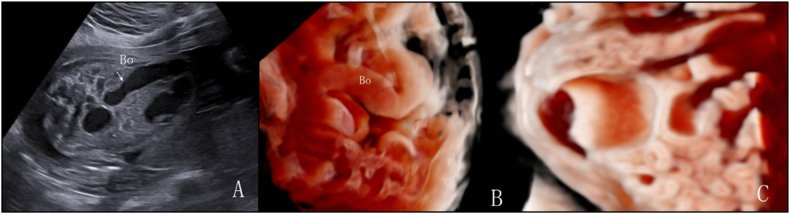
Fetus at 28 weeks and 5 days of gestation with small intestinal dilatation. Postnatal surgery confirmed distal jejunal atresia. A. 2D ultrasound shows dilated intestine with clear acoustic transmission through the intestinal contents. B. 3D imaging inversion mode demonstrates changes in the morphology of the dilated intestine. C. 3D imaging grayscale mode confirms the dilated intestinal segment as the small intestine with the smooth inner wall and the absence of haustra of colon.
